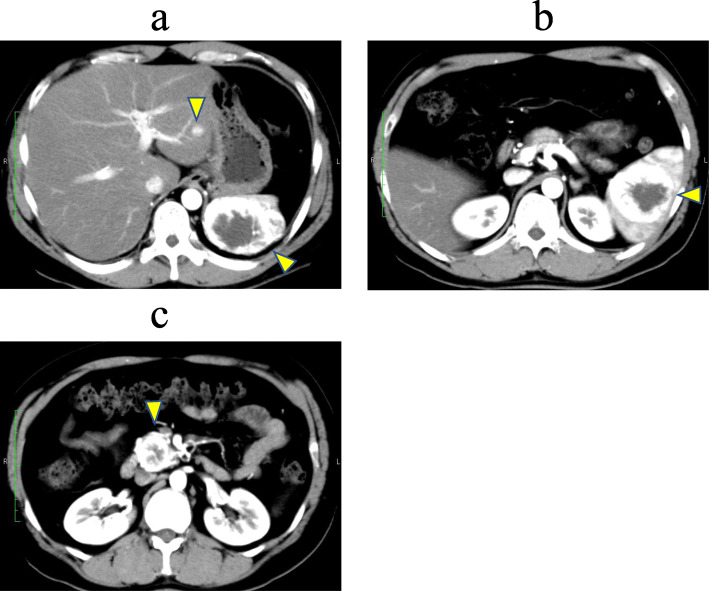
Contrast-enhanced computed tomography (CT) (horizontal slice). Contrast-enhanced CT revealed solitary lesions with enhancement in the liver (a), spleen (b), and pancreatic head (c) (arrow)
